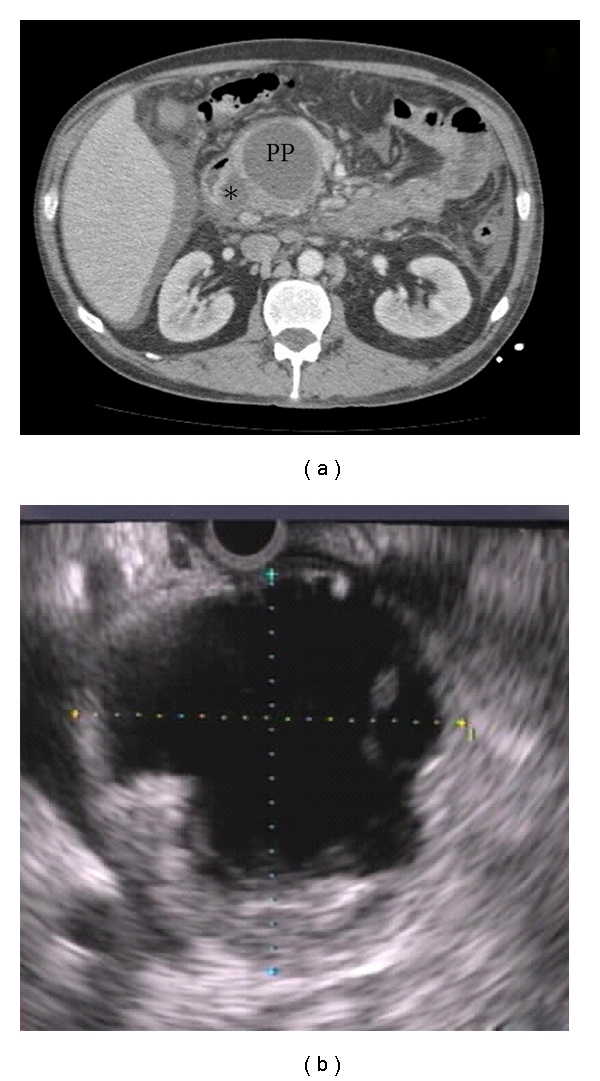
Pancreatic pseudocyst. (a) Typical appearance on CT, showing a dominant pancreatic pseudocyst (PP) with a smaller pseudocyst (asterisk) impinging slightly on the air- and fluid-filled duodenum. (b) Typical EUS appearance of a pseudocyst, with debris.
